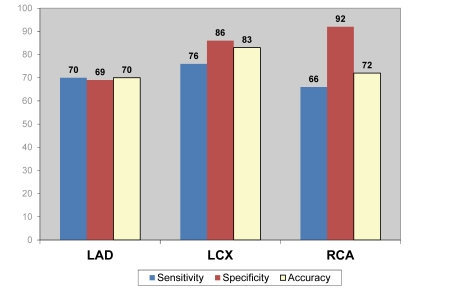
Pooled diagnostic value of PET/CT for detection of coronary artery disease based on individual coronary artery assessment.
